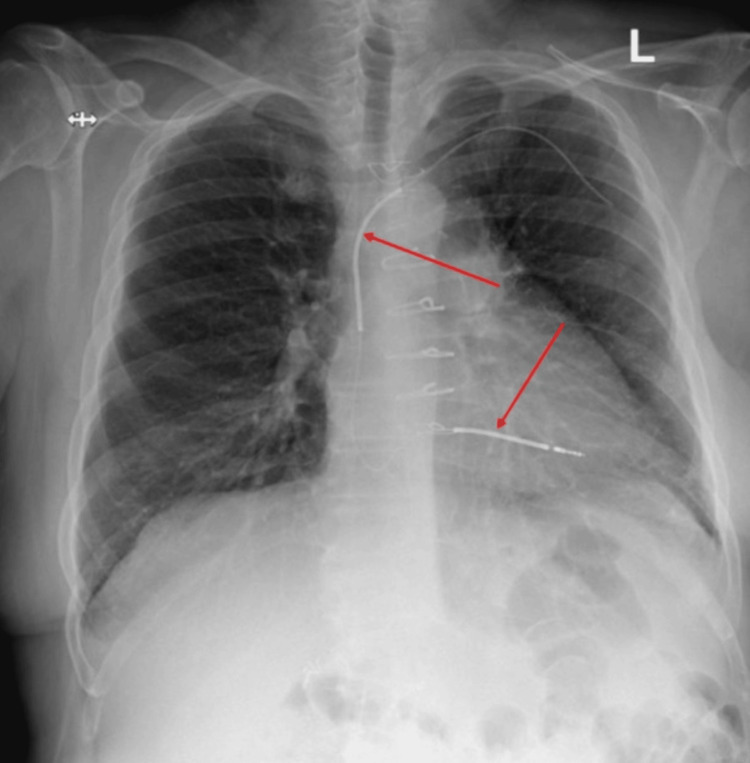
Posterior-Anterior (PA) chest radiograph demonstrating right ventricular implantable cardiac defibrillator lead (red arrows) but missing generator. Chest radiograph taken on day one of admission.
